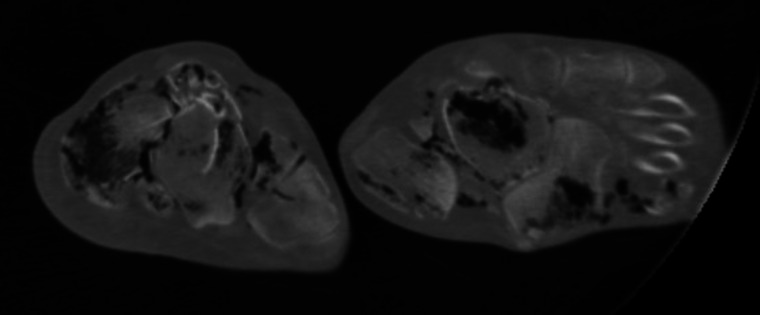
CT images showing comminuted diffuse fractures of the feet bones
